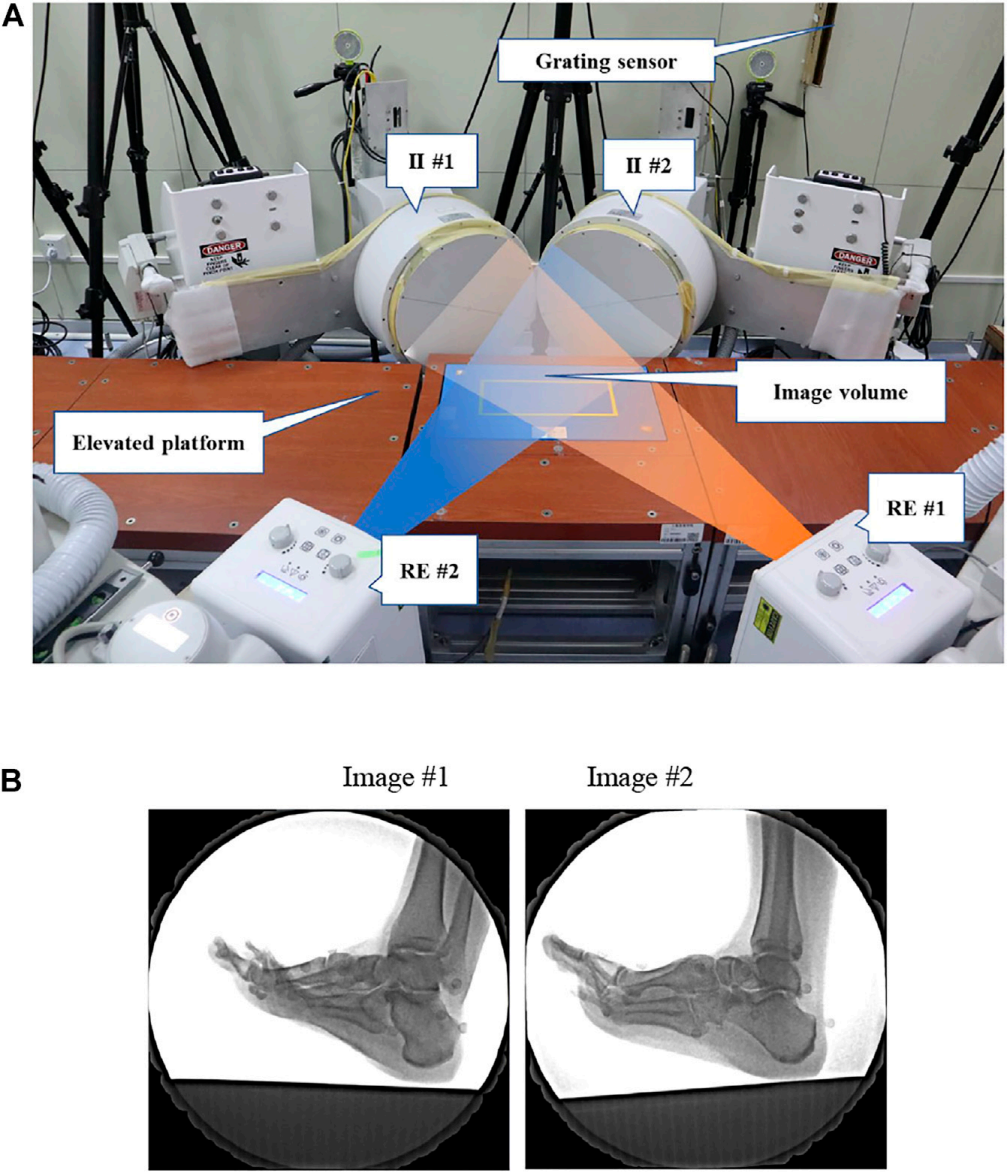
(A) High-speed DFIS setup. Participants ran on an elevated platform. The image intensifiers (II #1 and II #2) processed images created by X-rays from the radiographic emitters (RE #1 and RE #2). (B) DFIS images showed the first MTPJ of a participant.
